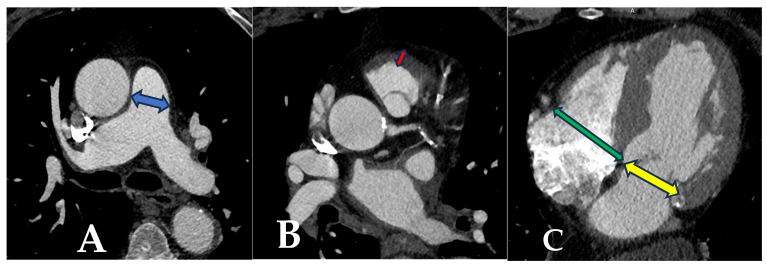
(A) Main pulmonary artery diameter was measured perpendicular to the vessel axis at the widest point. (B) The muscular thickness of the RVOT was measured anteriorly ~1 cm below the pulmonary valve. (C) Apical 4-chamber maximal right ventricular diameter and left ventricular diameter ratio.
